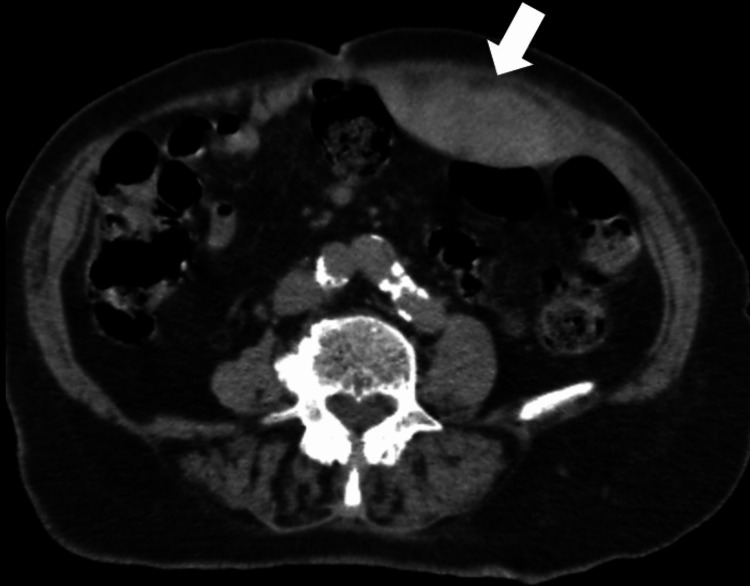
Left RSH (arrow) on axial CT image taken after USG on the same day (Patient 10)
